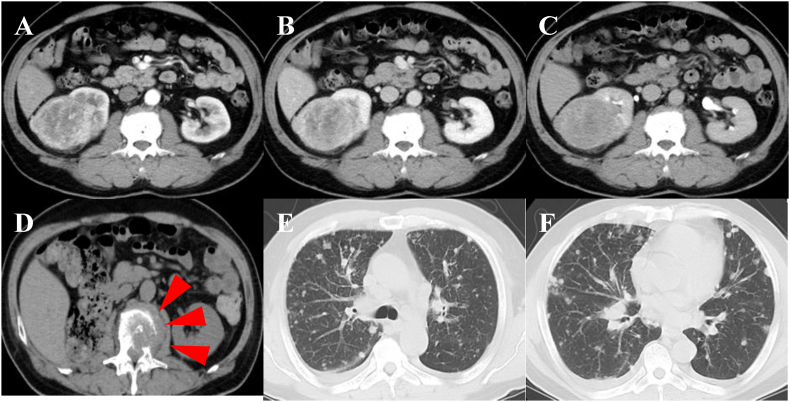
Computed tomography (CT) images of the abdomen and chest. A–C: Contrast-enhanced CT prior to surgery showing a right renal mass. A: 30 s. B: 70 s. C: 300 s. D: CT image of L2 bone metastasis upon the diagnosis of postoperative recurrence (red arrows). E, F: CT images of multiple lung metastases upon the diagnosis of postoperative recurrence.
